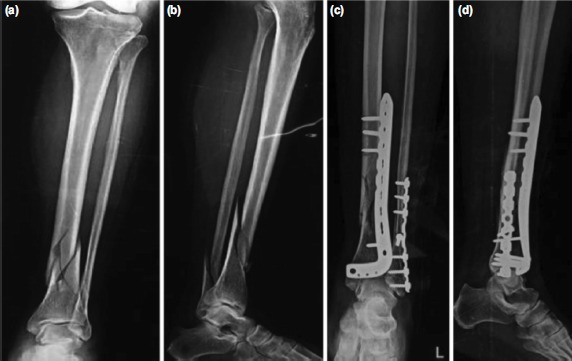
Pre-operative radiographs of extra-articular distal tibia fracture AO Type 43-B3 showing (a) antero-posterior and (b) lateral view. Immediate post radiographs showing ORIF with plating done in (c) antero-posterior and (d) lateral view.
